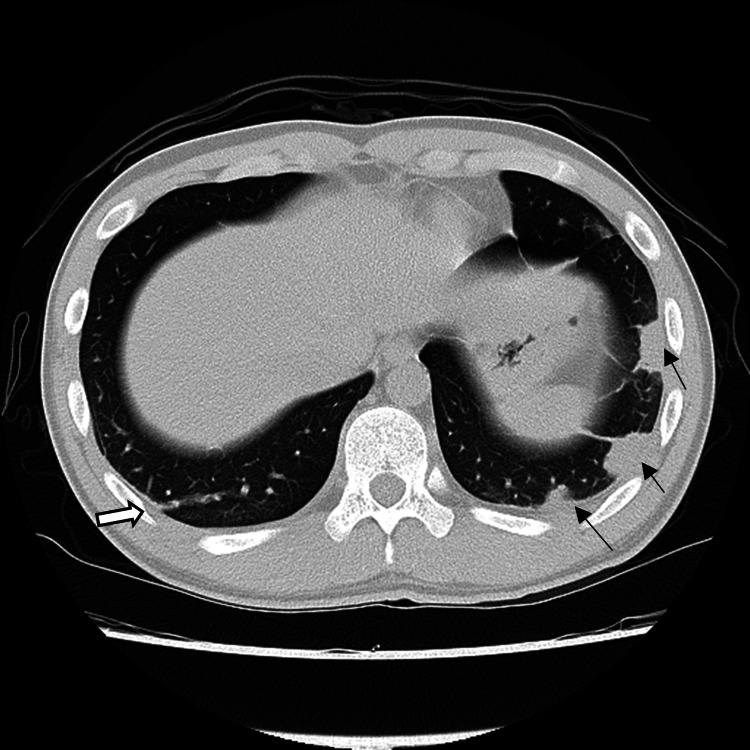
CT on day 18; changes due to septic pulmonary embolus or inflammatory nodules. →: Suspected septic pulmonary embolic changes or encapsulated pleural effusion ⇨: Changes due to septic pulmonary embolism or suspected inflammatory nodule
